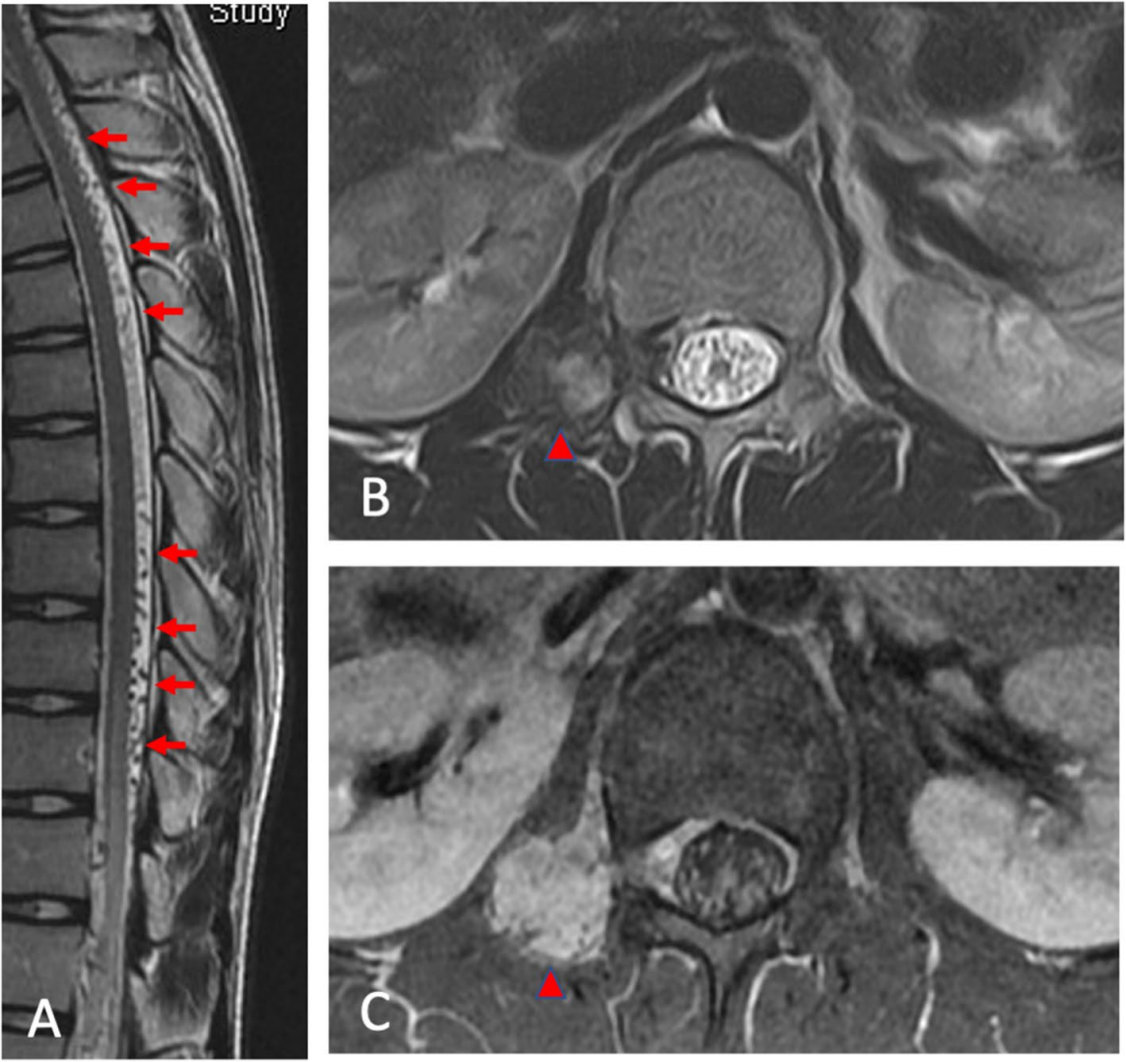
Sagittal spine MR T2-weighted image demonstrated flow voids at perimedullary spaces (A, arrows). Axial views at the level of right L1/L2 intervertebral foramen revealed the spinal dural arteriovenous fistula (SDAVF) arose around a circumscribed tumor which was heterogeneously hyperintense on T2-weighted images (B, arrowhead) and diffusely enhanced on post-contrast T1-weighted images (C, arrowhead)
